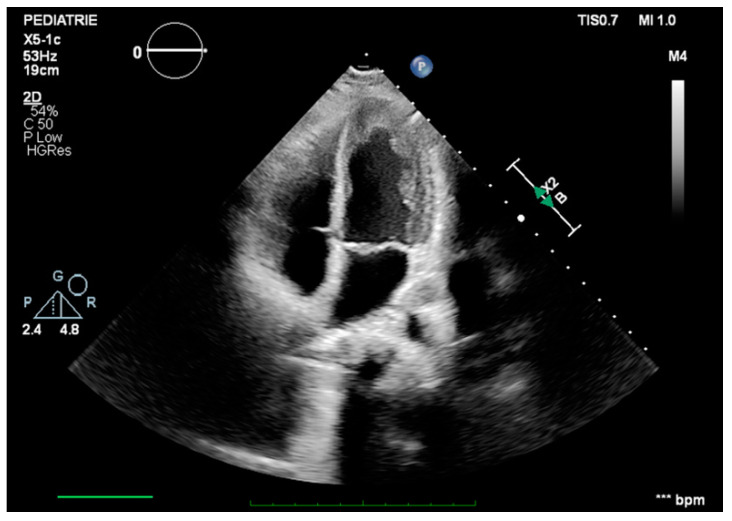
Apical four-chamber echocardiographic view during the early postoperative period, showing no residual tumor.
